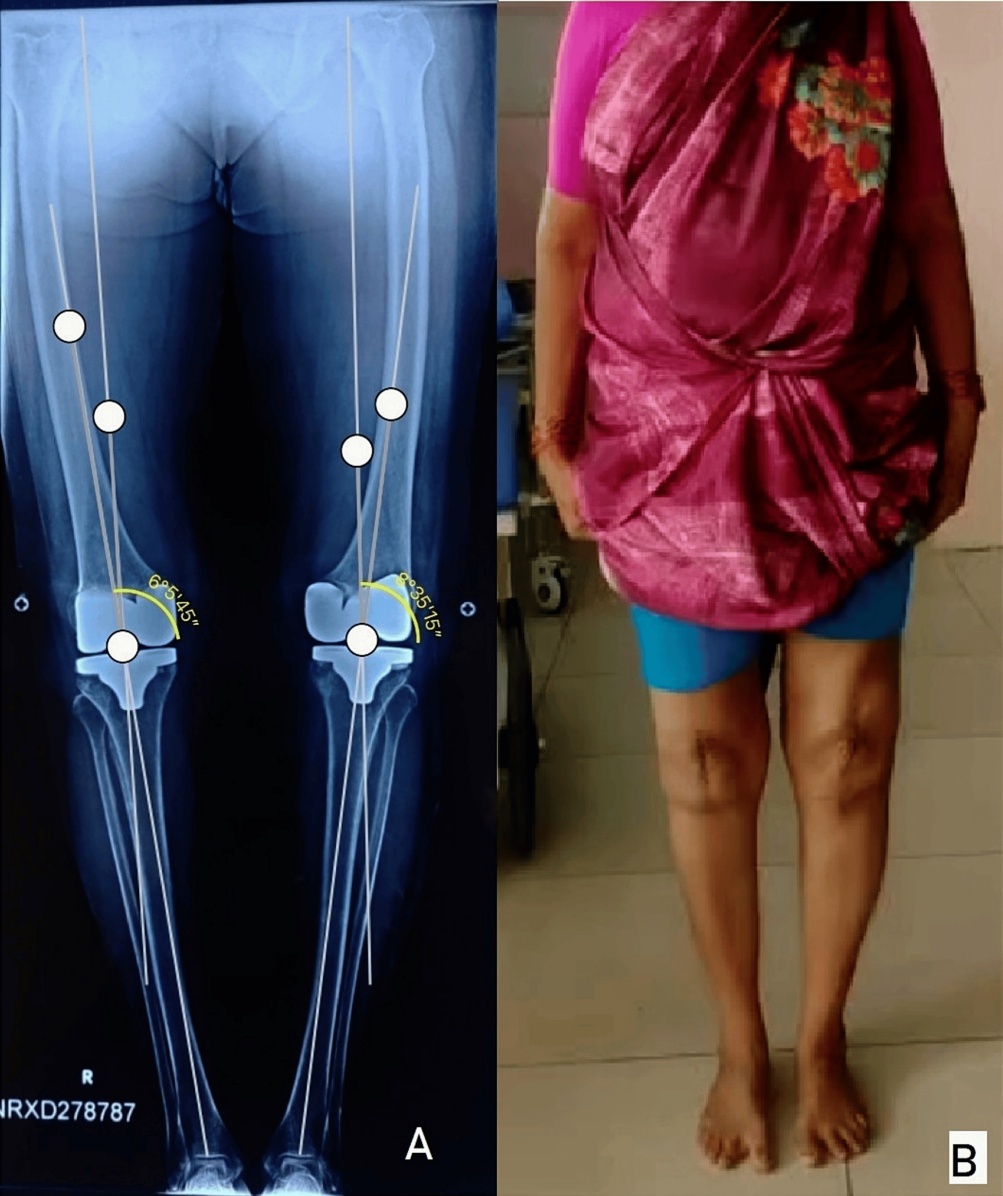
Postoperative full-length standing radiograph of bilateral lower limb (A) and clinical image (B) showing correction of deformity.
